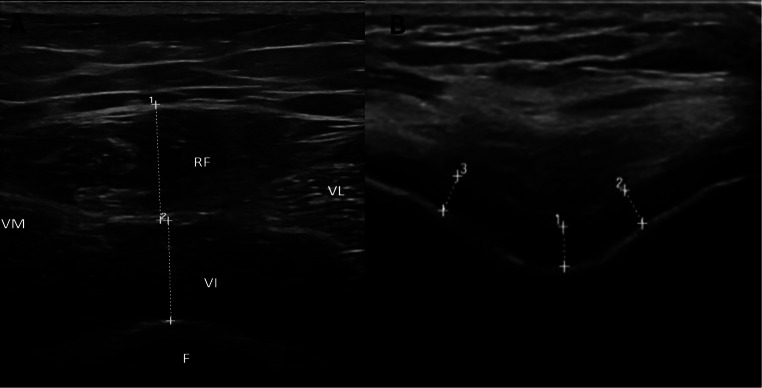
Ultrasonographic measurement of quadriceps and femoral cartilage thickness
